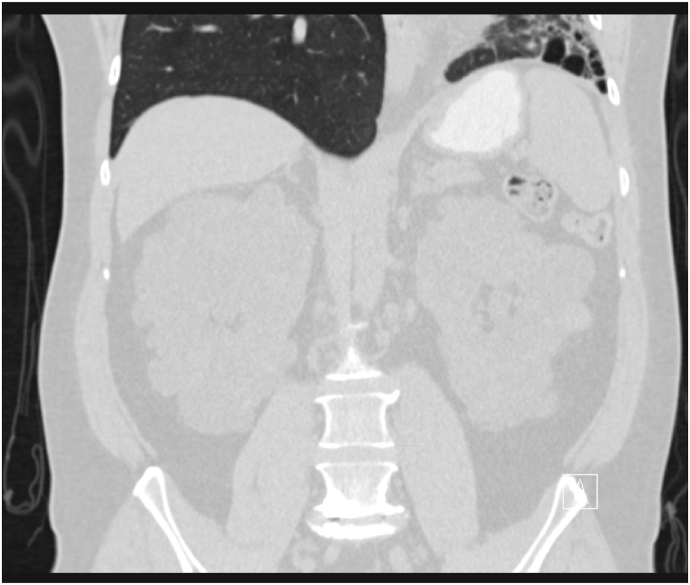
CT scan of the abdomen showing left lung base fibrosis and airspace disease as well as bilateral extensive kidney cystic disease consistent with patient's history of ADPKD.
